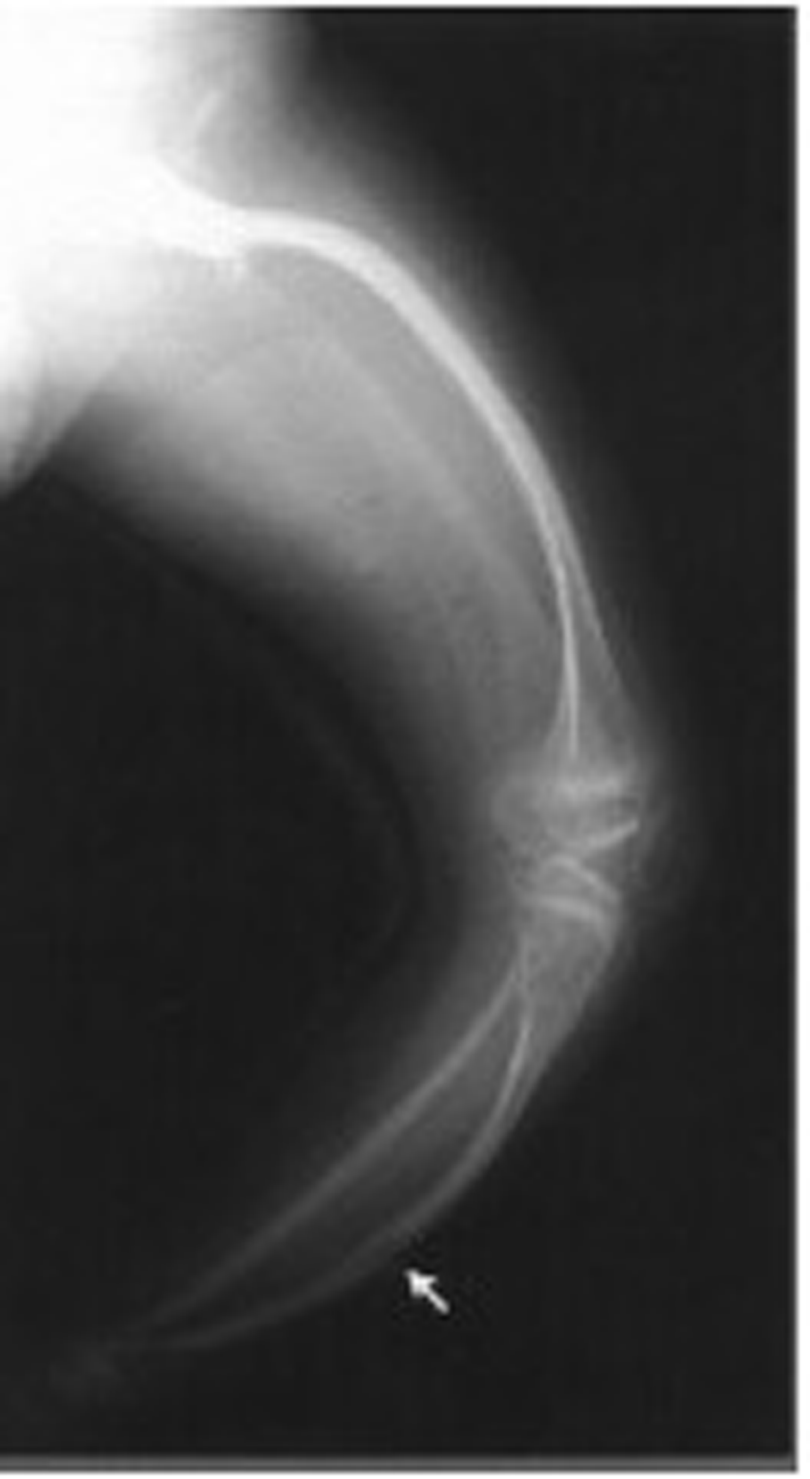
Femur and Tibia X-ray of 7 year-old female with MSS demonstrating dysplastic hips with shallow, horizontal acetabula, post-traumatic bowing, Arrow indicates healing tibial fracture. Diaphyses are gracile with thin cortices and obliterated medullae, in contrast with widened metaphyses and epiphyses, which are relatively spared [13].
